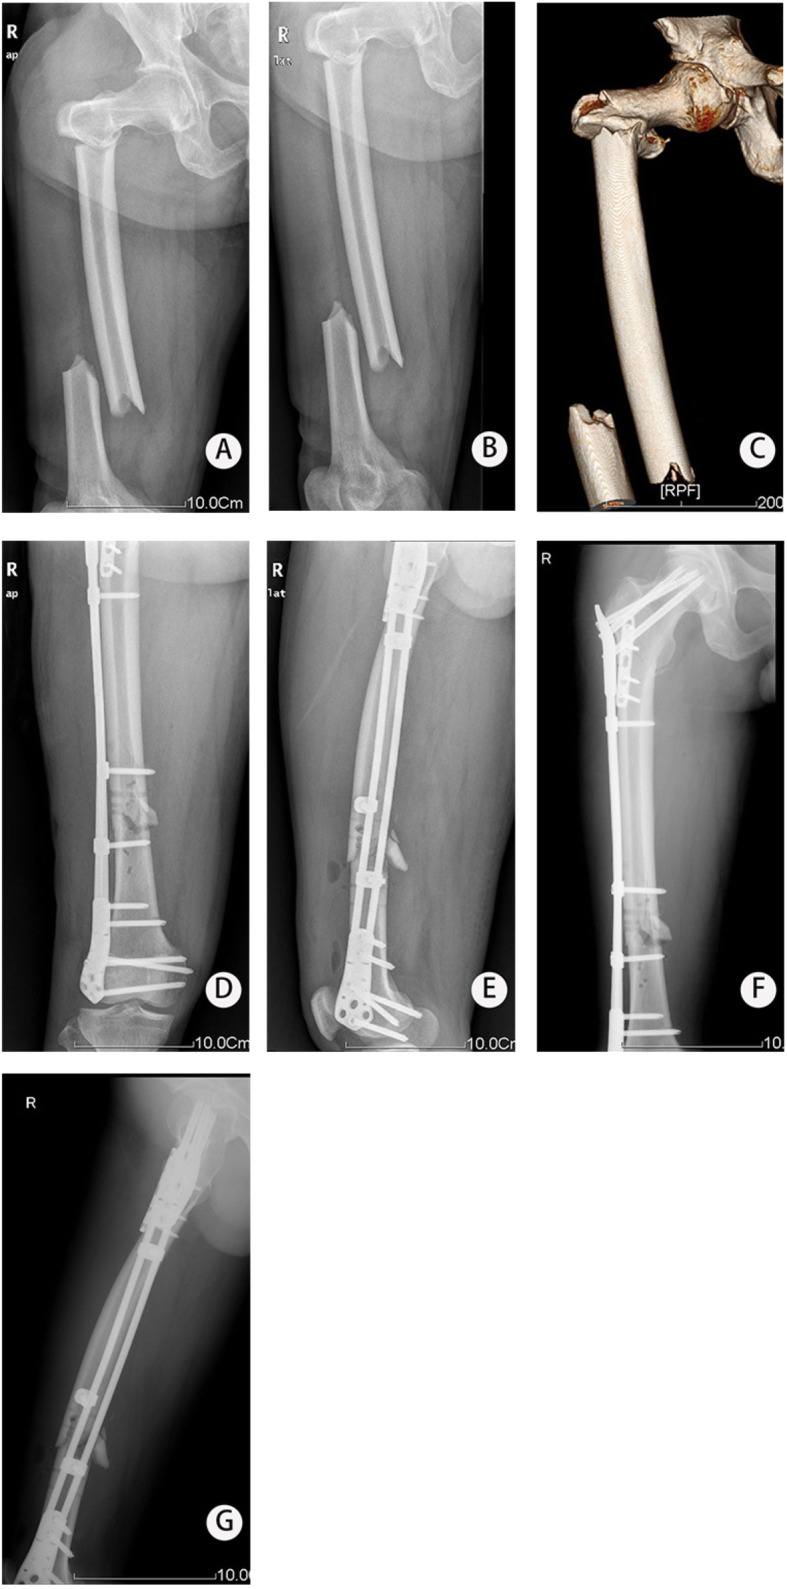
Representative images of patient 12 who lost connection at 3-month follow-up. a–c X-ray at admission. d–i X-ray at 3 days after operation
